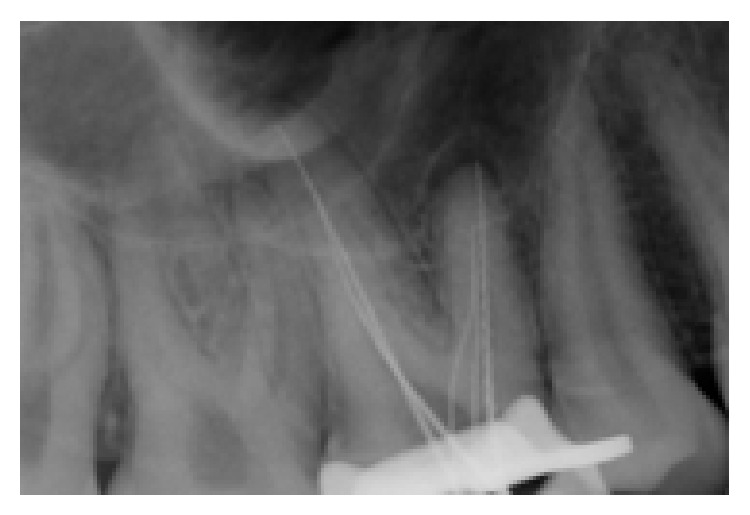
Working length radiograph showing three MB canals. MB3 joining the MB2 at middle third.
